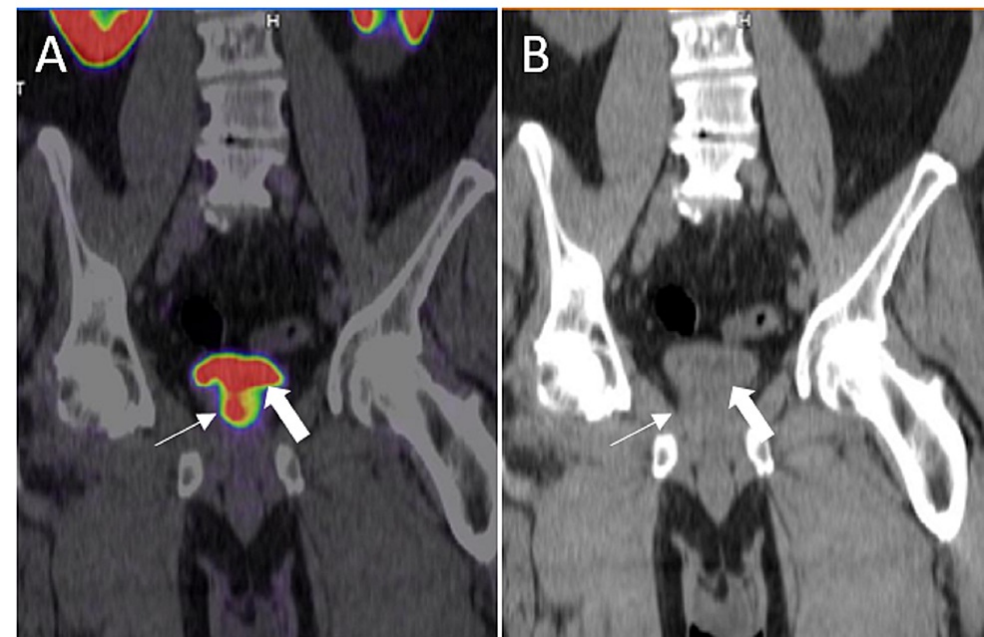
Urinary bladder invasion A: Coronal 68Ga PSMA PET/CT shows primary prostate cancer (white thin arrow) invaginating to the urinary bladder (white thick arrow). B: Correlate coronal CT shows the prominent prostate (white thin arrow) and absent fat plane between the prostate and the urinary bladder (white thick arrow).
